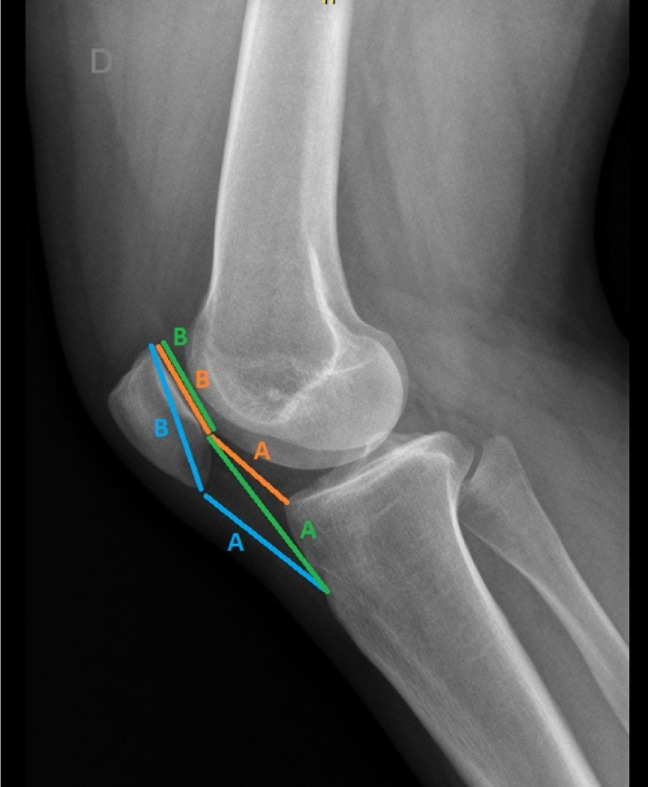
Radiograph demonstrating the conventional ratios on a lateral knee: IS (blue), mIS (green), and C-D (red). The IS ratio was calculated by dividing the length of the patellar tendon with the longest diagonal distance between the lower and the upper end of the patella. A value higher than 1.20 indicated PA. The mIS ratio was calculated by dividing the distance between the patellar tendon insertion and the lower end of the articular surface of the patella with the length of the articular surface of the patella. Values higher than 2.00 indicated a PA. The C-D ratio was calculated by dividing the distance between the peak of the visualized anterosuperior angle of the tibial plateau and the lower end of the articular surface of the patella with the length of the articular surface of the patella. Values higher than 1.30 indicated PA. C-D = Caton-Deschamps, CE = clinician's eye, IS = Insall-Salvati, mIS = modified Insall-Salvati, PA = patella alta.
